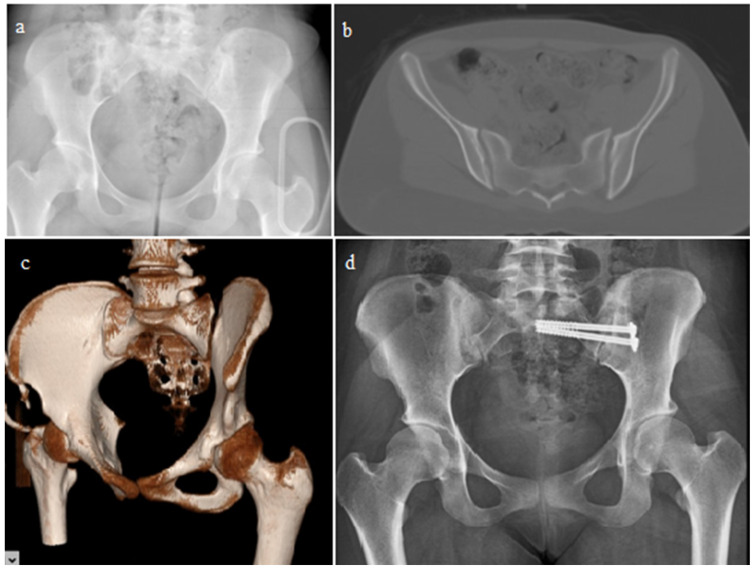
A 32-year-old woman with pure sacroiliac joint dislocation. Two screws were applied percutaneously. Pelvic radiography (a), tomography images of the pelvis (b,c), and postoperative third-year pelvic radiography (d).
